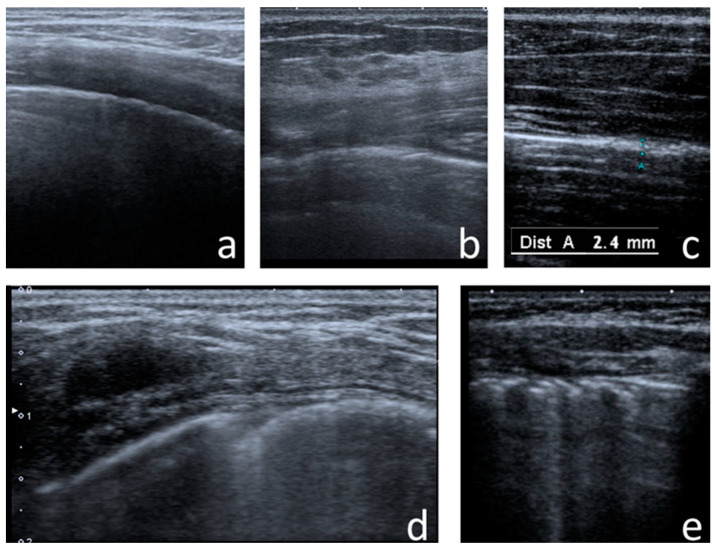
Abnormal pleural line: (a) irregular; (b) blurred; (c) thickened; (d) ragged (some sonographers would describe a small consolidation in this case); (e) fragmentary.
